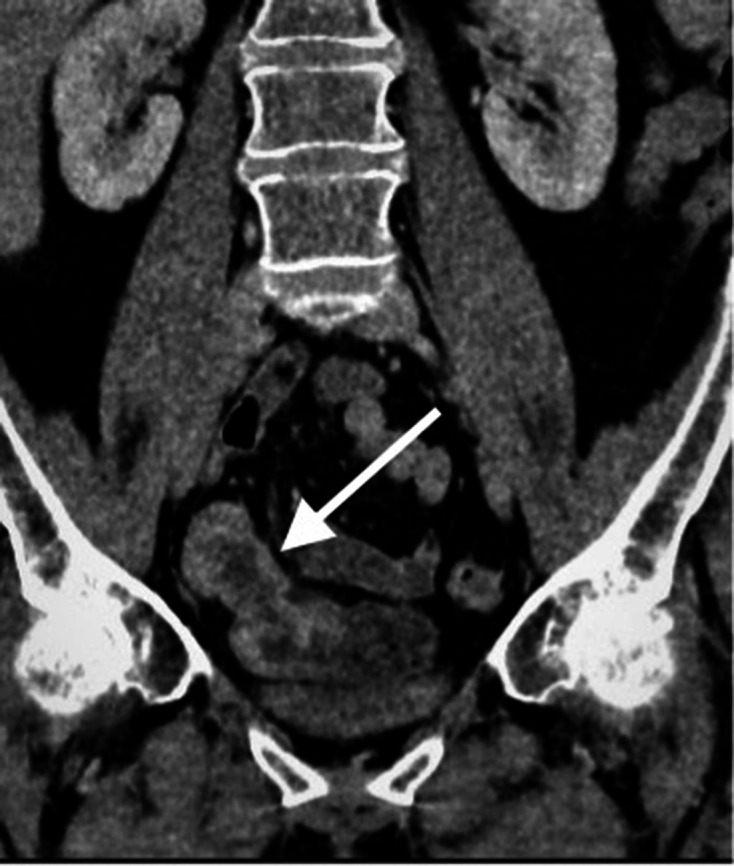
Contrast enhanced CT scan of the abdomen and pelvis in coronal plain
